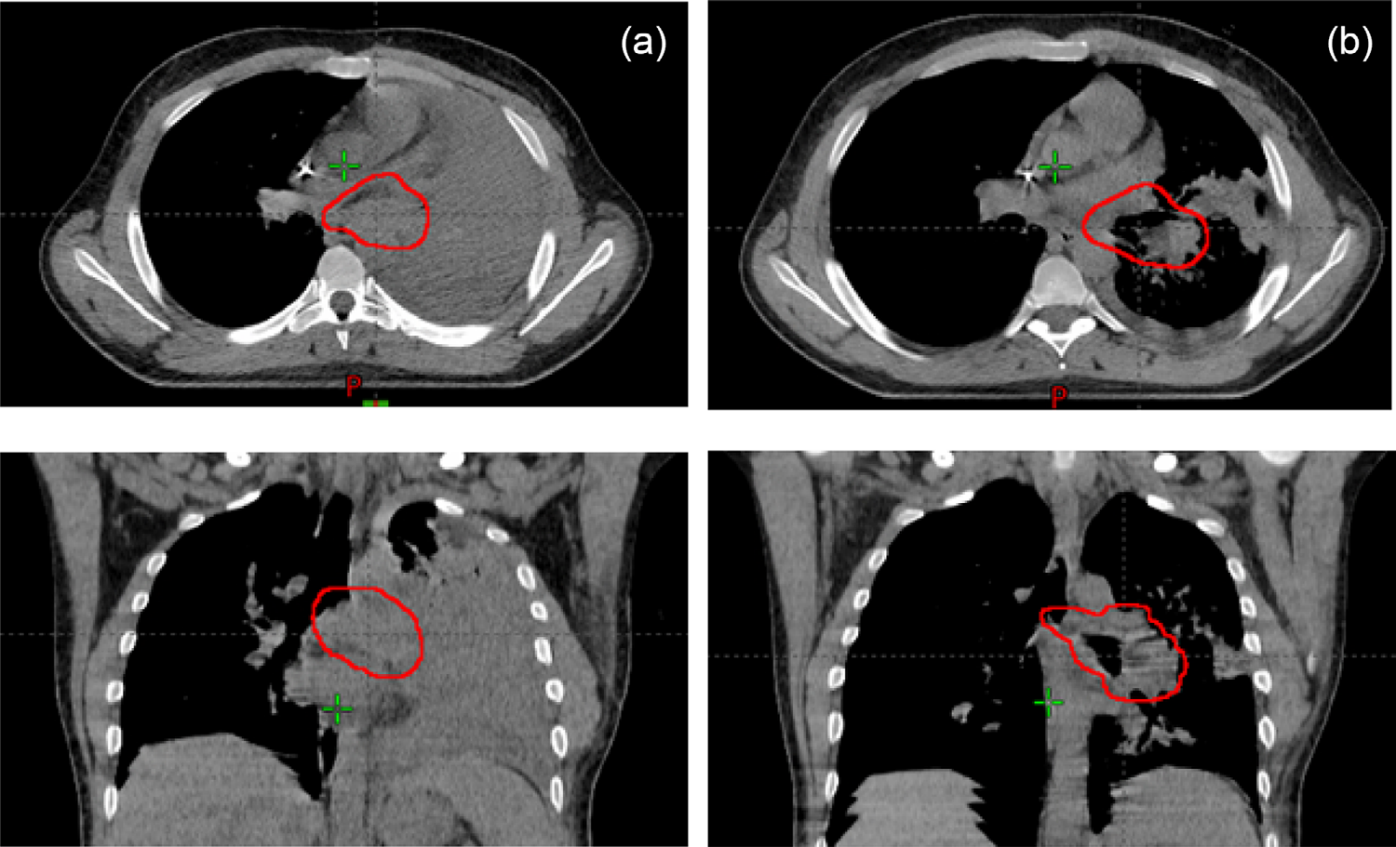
(a) Initial planning CT with the PTV contoured in red and (b) planning CT after five treatment fractions.
